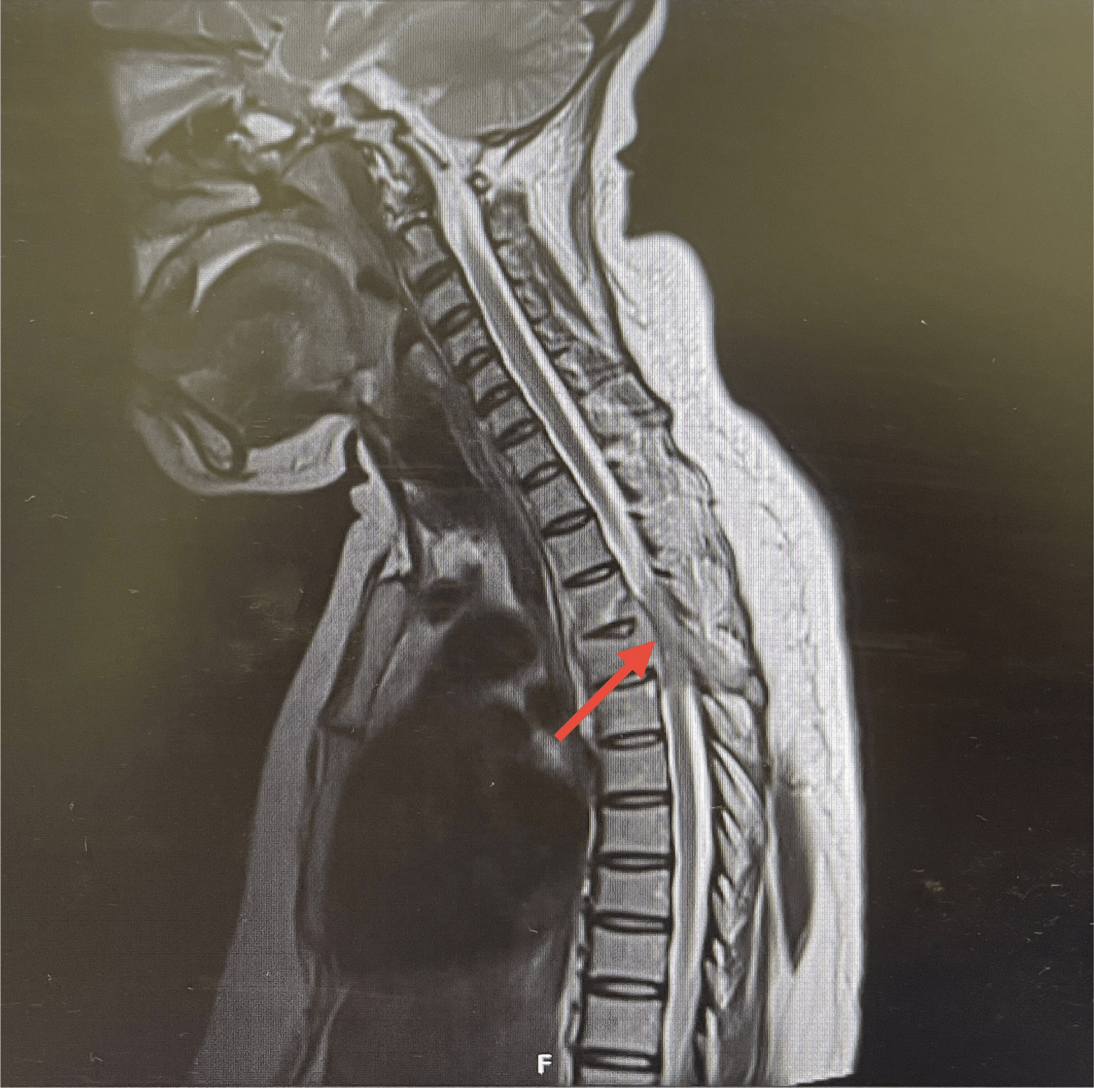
Pre-treatment MRI showing destructive changes involving T3-T5 vertebral bodies with significant T4 spinal cord compression (arrow).
